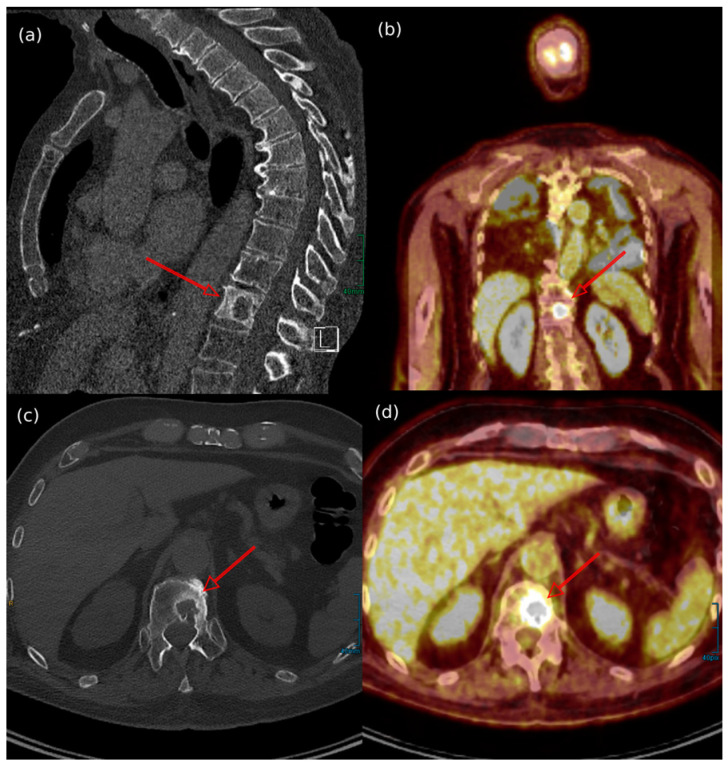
Osteolytic bone metastasis from Merkel cell carcinoma. Sagittal (a) and axial (c) CT images show an osteolytic lesion in the D10 vertebral body (red arrows). Corresponding coronal (b) and axial (d) PET/CT images demonstrate FDG uptake in the same lesion (red arrows). No gross epidural involvement is observed in this patient.
